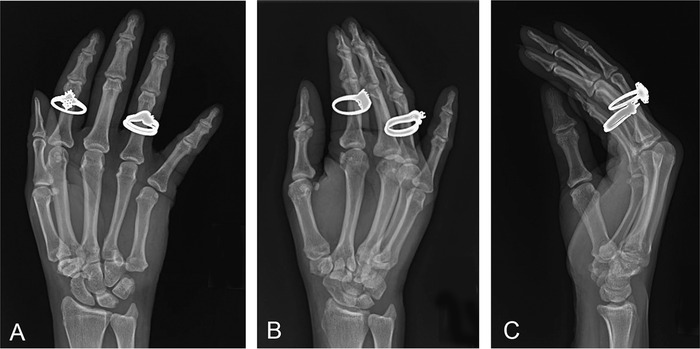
Right hand posteroanterior (A), oblique (B), and lateral (C) x-rays at initial presentation in the emergency department. Fifth carpometacarpal joint dislocation was not identified.
